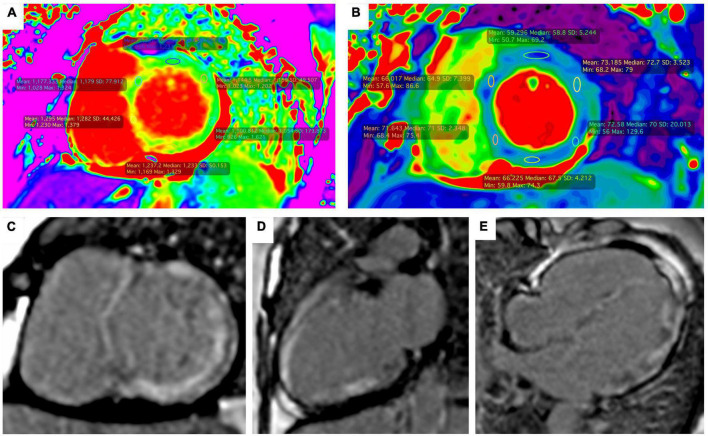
Magnetic resonance imaging. Short-axis ShMOLLI color maps showing diffuse high native values T1 (A) and T2 (B) which is compatible with myocardial edema and fibrosis. Late gadolinium enhancement with intramyocardial and transmural pattern was observed in the short axis view (C), two chambers view (D) and four chambers view (E).
